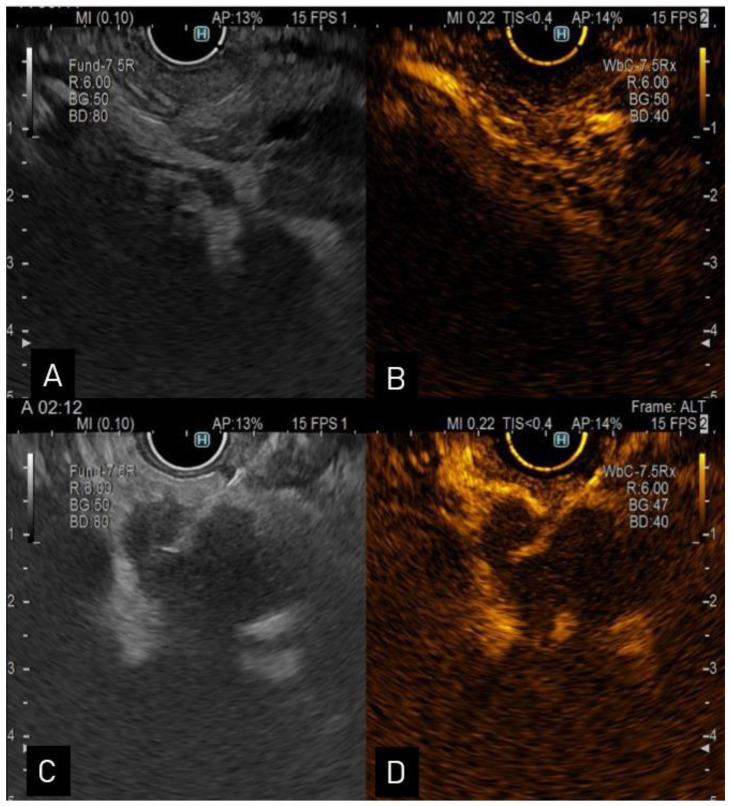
(A). Endoscopic ultrasound view of a distal CCA; (B). contrast enhanced-ultrasound showing hyperenhancement in the arterial phase; (C). endoscopic ultrasound view of a proximal hypoechoic bile duct lesion undergoing aspiration via a 22-G needle; (D). contrast enhanced-ultrasound guided aspiration.
